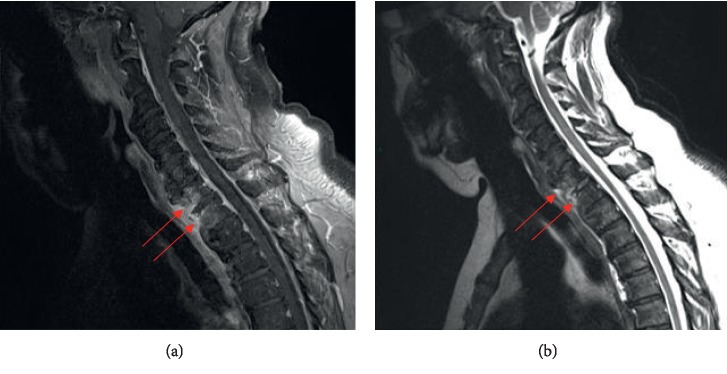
MRI of the cervico-dorsal junction, T1-weighted post-gadolinium injection (a) and T2-weighted (b) sequences, showing enhancement in C7 and T1 vertebral bodies after injection, and hyperintense signal on T2 sequence (arrows). The intervertebral C7-T1 disc does not show any T2 hypersignal or enhancement following contrast injection.
